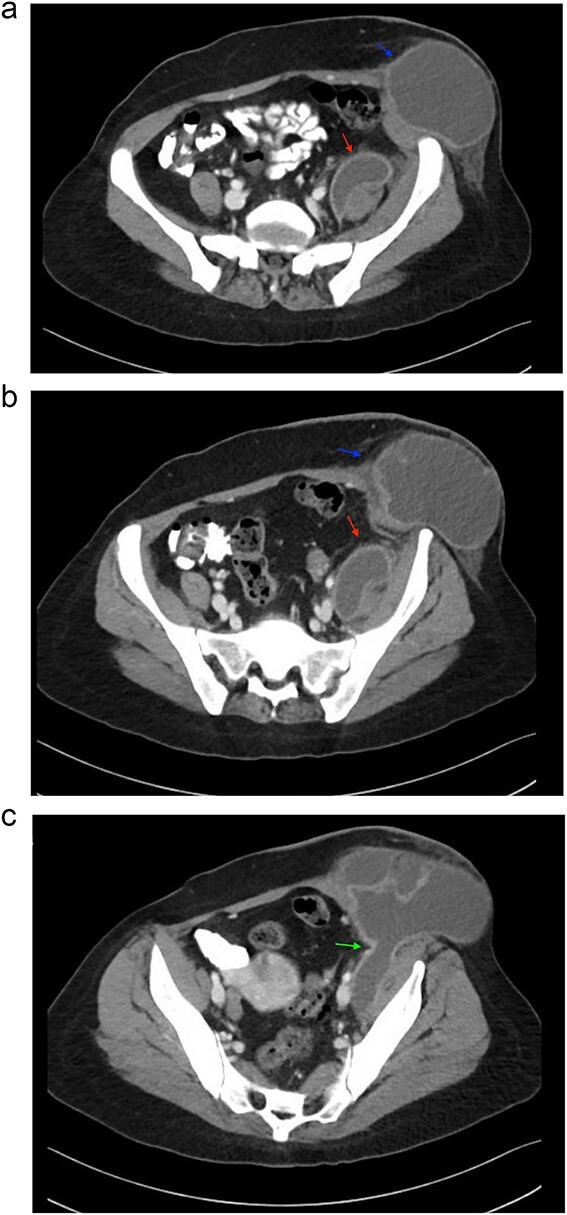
Sequential images of CT with contrast of the abdomen and pelvis demonstrating the pathology. (a) Slice 96—a small fluid collection in the left psoas muscle (lower arrow), and a low-density fluid collection in the left inguinal region (upper arrow), both with wall enhancement, consistent with abscess formation. (b) Slice 102—progression and enlargement of the iliopsoas (lower arrow) and inguinal (upper arrow) abscesses. (c) Slice 110—connection between the iliopsoas and inguinal abscesses, indicating advanced spread of infection (arrow).
